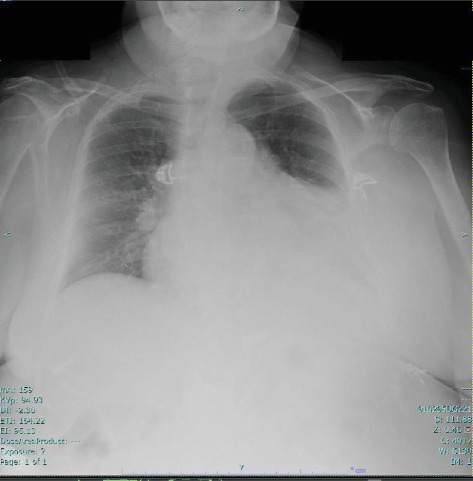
Chest X-ray shows a left lower lobe pneumonia associated with a parapneumonic effusion.
